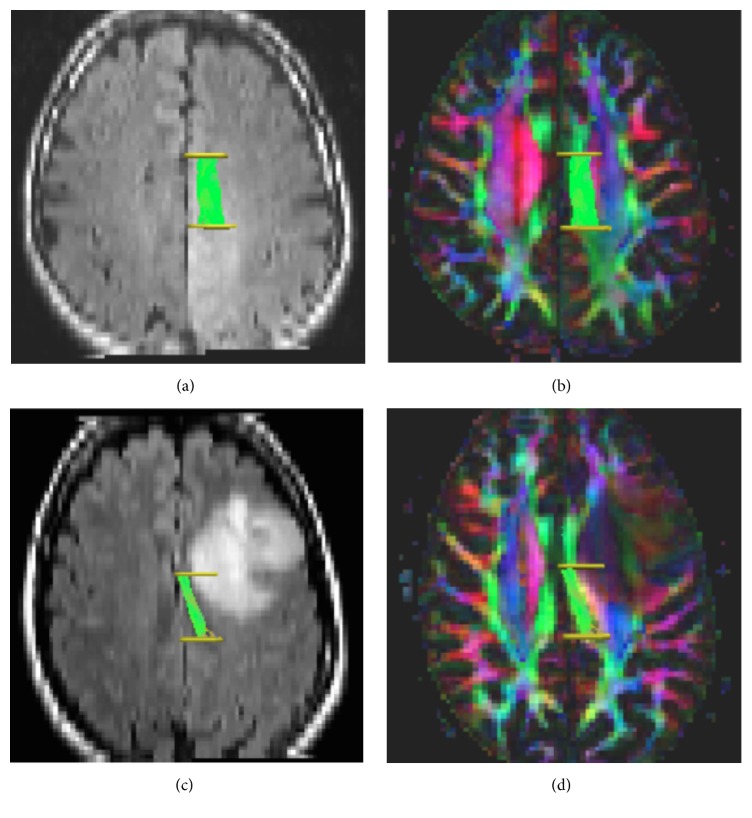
Tractography of segment 2 of the cingulum in two different patients (patient number 14 and patient number 25). ((a)-(b)) Patient with an astrocytoma grade III. (a) 3D-tractography of segment 2 of the cingulum overlaid on T2-FLAIR image shows tumor infiltrating the area of the tract segment. ROIs number two and three in yellow. (b) 3D-tractography in the same patient, segment two of the cingulum overlaid on 2D FA-color map. ((c)-(d)) Patient with an oligodendroglioma grade II. (c) 3D-tractography of segment 2 of the cingulum overlaid on T2-FLAIR image shows tumor dislocation of the tract segment. ROIs number two and three in yellow. (d) 3D-tractography in the same patient, segment two of the cingulum overlaid on 2D FA-color map.
